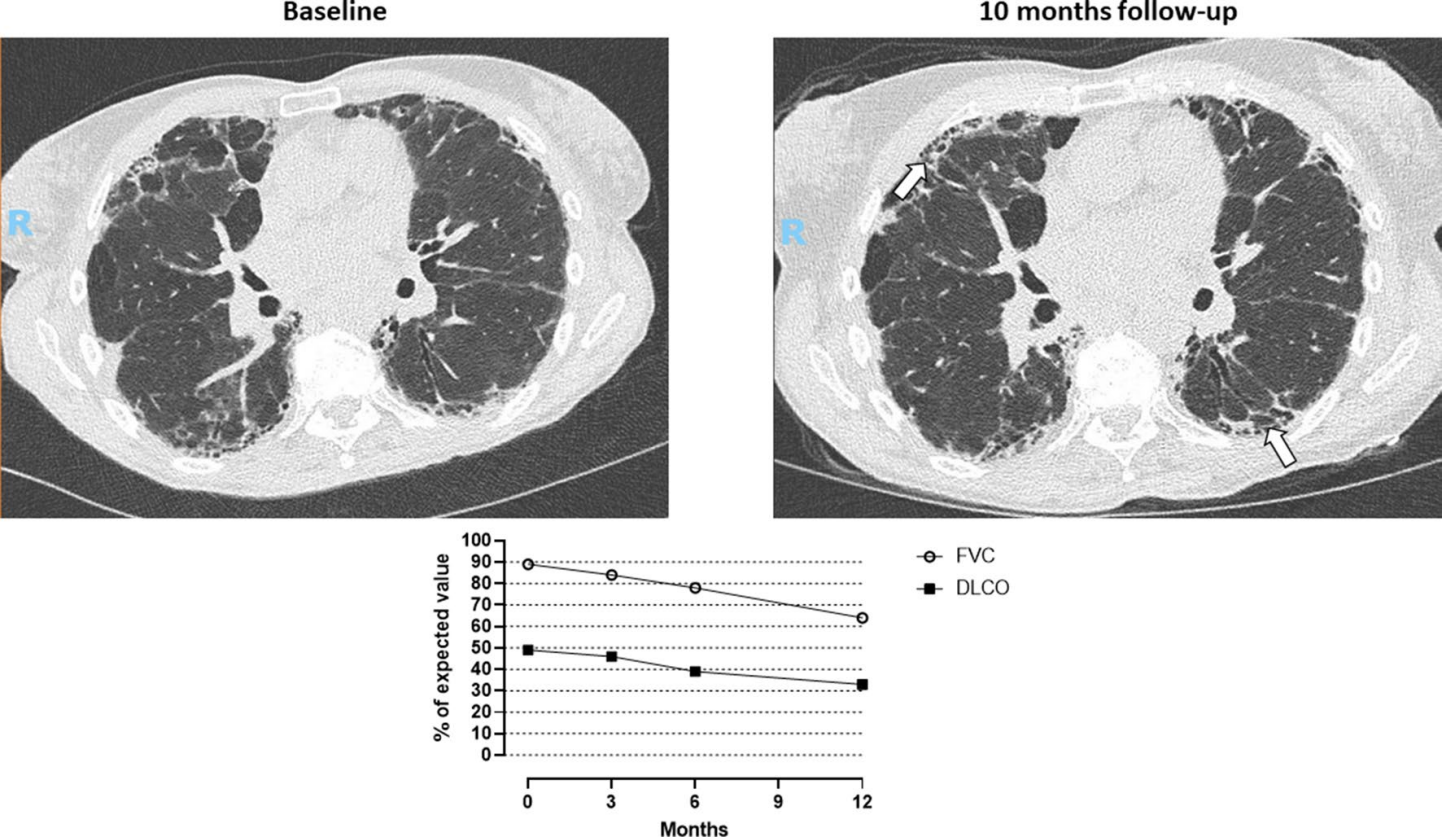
Example of a patient suffering from fibrosing hypersensitivity pneumonitis. 10 month follow-up high-resolution CT scan shows progression of fibrosis with honeycombing (white arrows). Longitudinal pulmonary function tests demonstrate decline of both forced vital capacity and lung diffusion capacity. FVC: forced vital capacity; DLCO: lung diffusion capacity.
